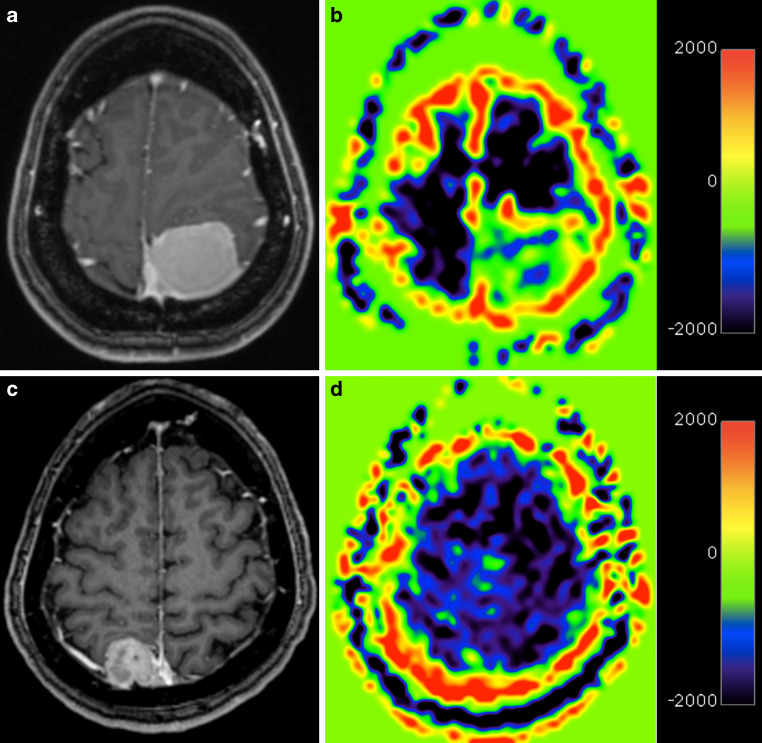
T1-weighted post-contrast images (a, c) and relative cerebral blood flow maps obtained by pulsed arterial spin labeling (b, d) of a 41-year-old patient with a meningothelial meningioma (a, b) and a 52-year-old patient with a dural metastases from breast cancer (c, d)
